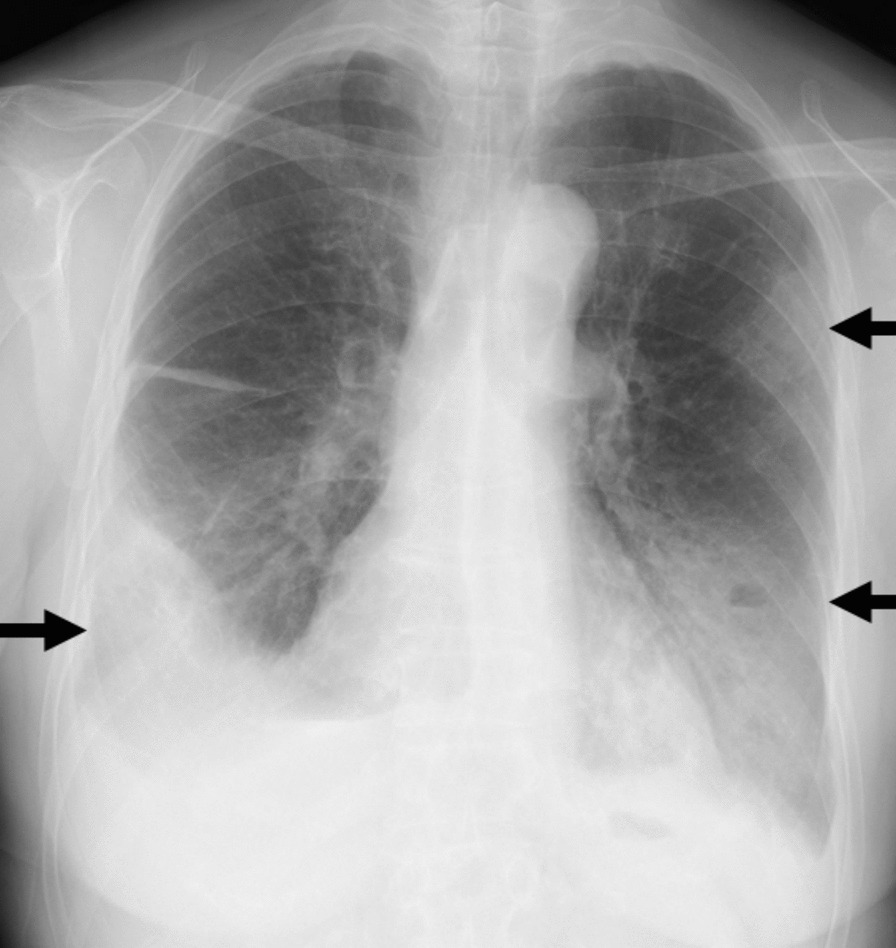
Chest radiograph at presentation revealing bilateral consolidation with an air–fluid level (black arrows)
